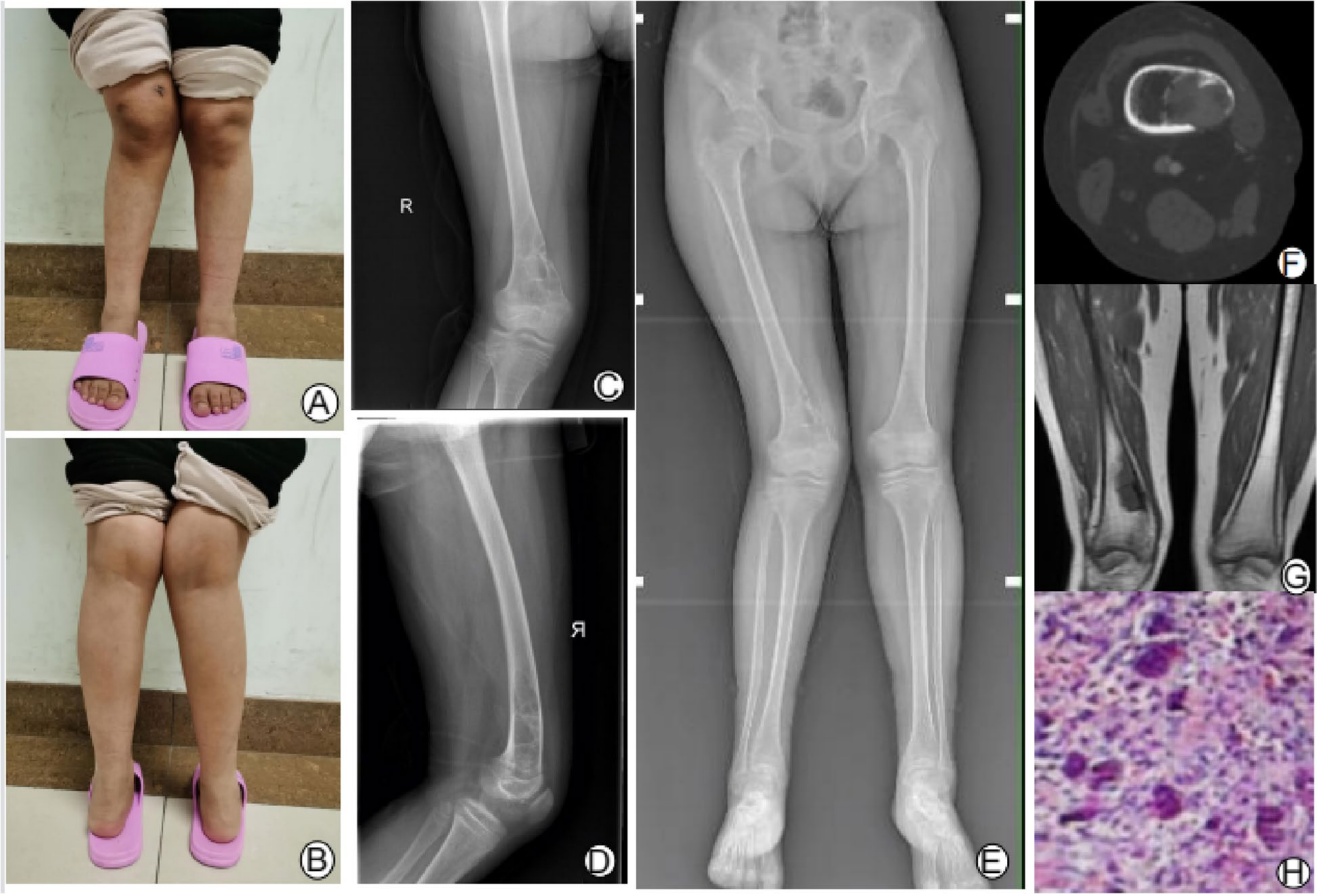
Ⓐ-Ⓑ Genu valgum deformity of the right lower limb. Ⓒ-Ⓔ X-ray showed partial osteolytic destruction of the metaphyseal segment of the femur, destruction of the medial cortex, and valgum deformity of the knee. X-ray of the whole length of the lower limb showed valgum deformity of the right knee. Ⓕ CT showed partial osteolytic destruction and cortical destruction of the femur, with clear boundaries and sclerotic edges. Ⓖ T1 imaging showed a low signal in the medullary cavity at the metaphyseal segment of femoral with clear boundaries. Ⓗ Spindle cell lesions and more multinucleated giant cells were observed in the preoperative puncture pathology images
